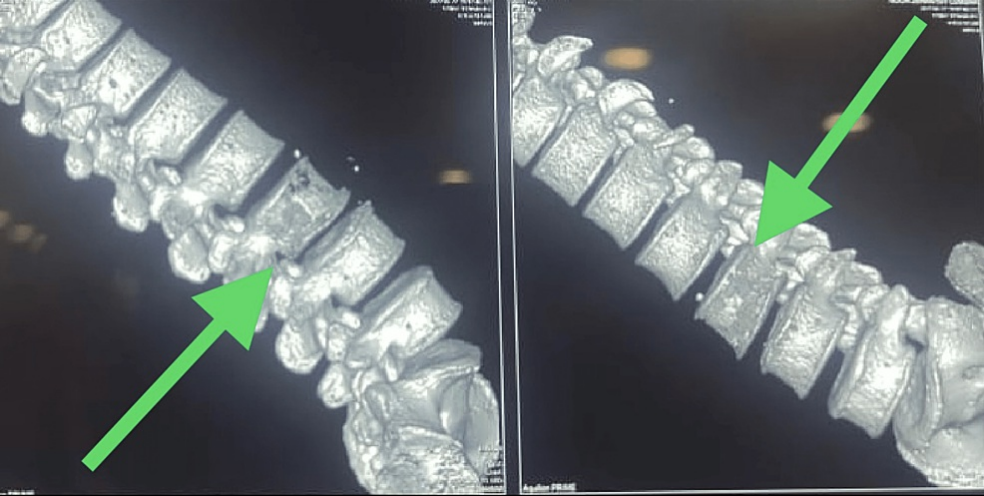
CT scan of the spine in the lateral plane view shows intraosseous air foci at the L3, indicated by the arrow. CT: computed tomography, L3: third lumbar spine vertebra
